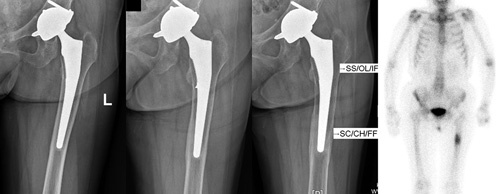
Serial radiographs of an 80-year-old woman with a 2-year moderate left anterior mid-thigh ‘start-up’ pain after uncemented total hip arthroplasty 7 years before. (A) A 4-year postoperative anteroposterior film showed no real radiolucency or distal femoral cortical hypertrophy (DFCH). (B) A 5-year postoperative film showed only a trace of DFCH. (C) Seven years after operation, there were extensive periprosthetic radiolucency in Gruen zones 1, 2, 6, and 7 and obvious DFCH in Gruen zones 3 and 5. (D) Technetium-99 methylene diphosphonate bone scintigraphy showed an increased uptake around the region of prosthesis stem tip. Inhomogeneous stress distribution [proximal stress shielding (SS) and distal stress concentration (SC)] after THA can cause periprosthetic femoral remodeling [proximal osteolysis (OL) and distal cortical hypertrophy(CH)] and subsequent possible periprosthetic stress fracture [proximal insufficiency fracture (IF) or distal fatigue fracture (FF)].
